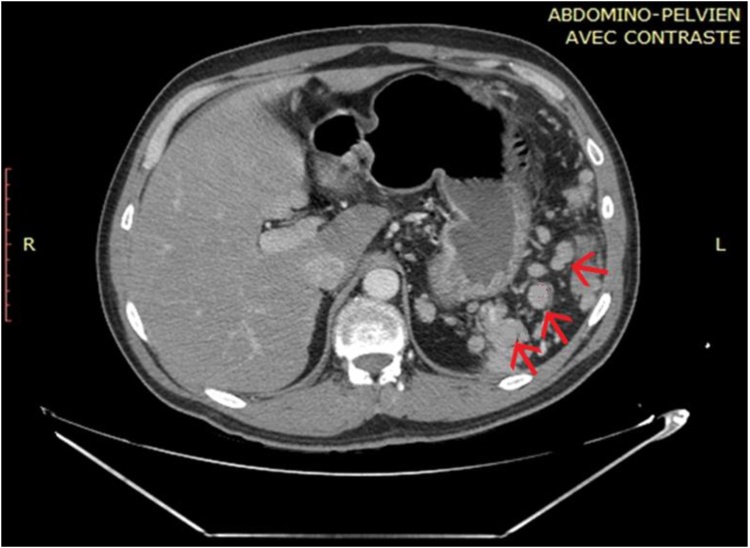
CT scan showing several splenic nodules in left upper quadrant and on the mesentery of the bowels (red arrows).
